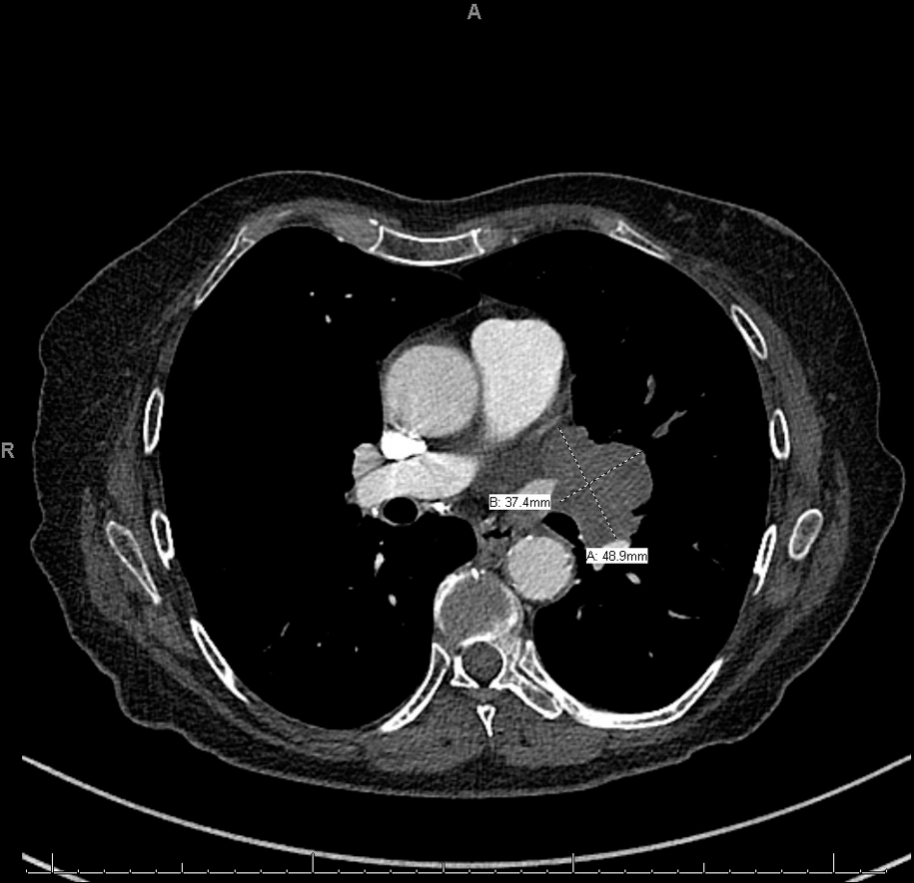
Chest computed tomography revealed a left perihilar mass measuring 4.9 × 3.7 cm with significant narrowing of the upper portion of the left lobe bronchus and pulmonary vein and narrowing of the lingual pulmonary artery. The mass was abutting the left main pulmonary artery and the left atrial appendage.
